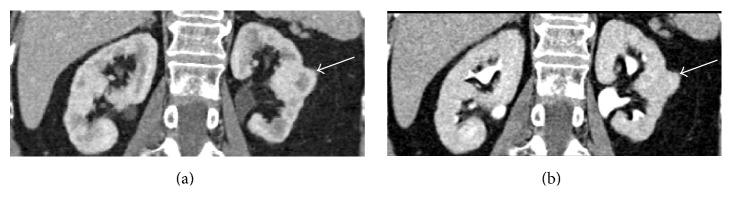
Hypertrophied column of Bertin. Coronal reformatted contrast-enhanced CT images in corticomedullary (a) and nephrographic phase (b) well demonstrate the enlarged column of Bertin (arrows) characterized by the same pattern of enhancement of the normal renal cortex.
